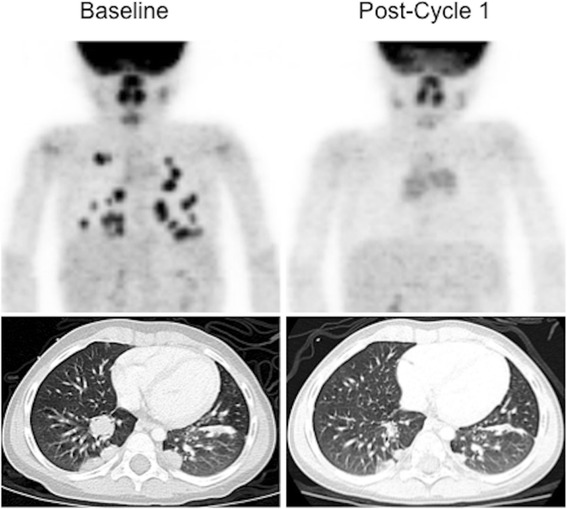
PET response to targeted experimental Phase 1 therapy: Crizotinib in ALK^+^ IMFT. ^18^F-FDG-PET and CT imaging of Crizotinib response in IMFT: Baseline whole body ^18^F-FDG-PET and Chest CT show multiple FDG-avid pulmonary nodules, confirmed by biopsy to be IMFT. Following 1 cycle of therapy, no residual abnormal FDG accumulation is seen (metabolic CR). The lesions have decreased significantly in size by CT, meeting criteria for partial reponse (PR), but not CR. This patient has remained free of disease for >36 months [32].
